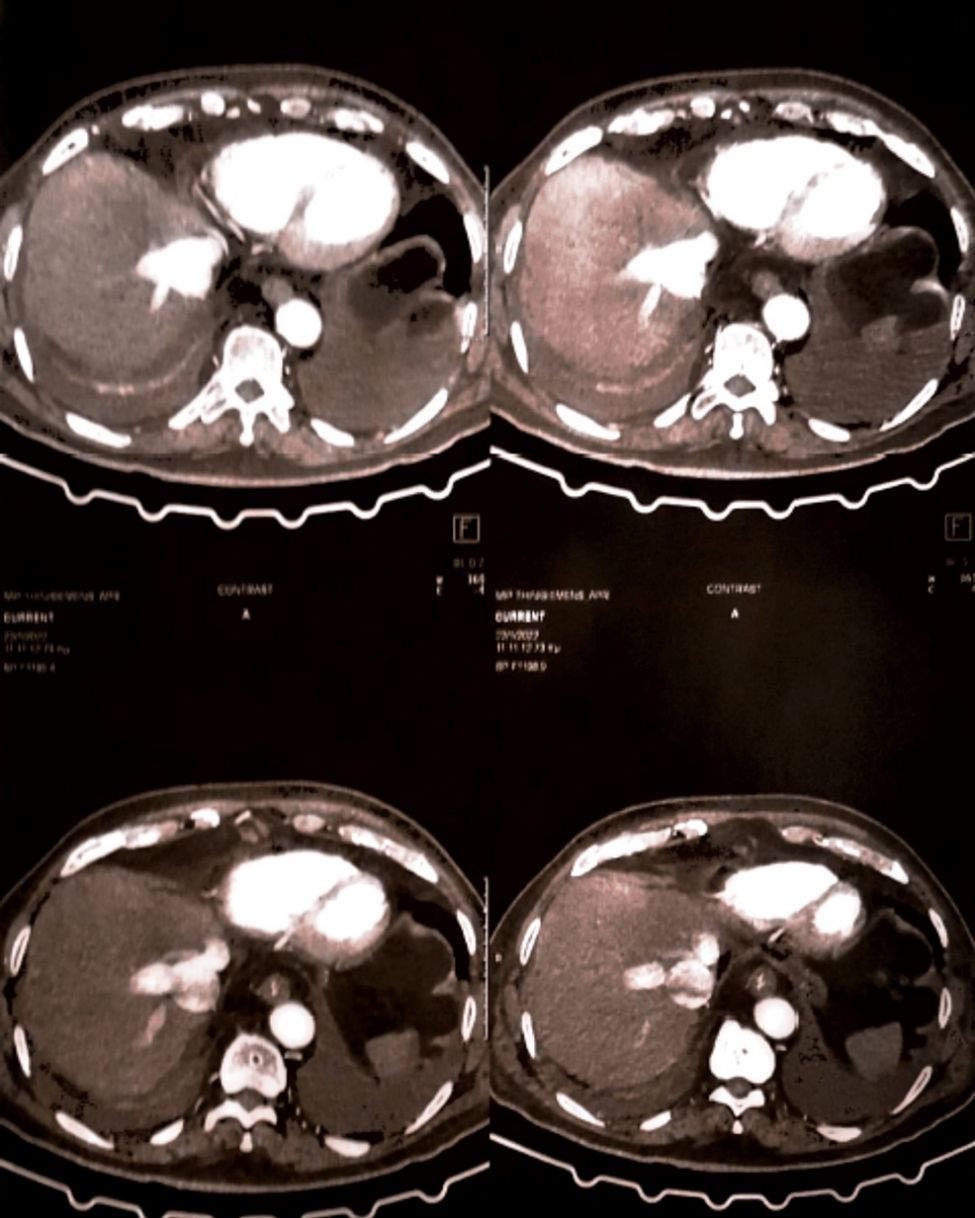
Computed tomography demonstrating mild liver enlargement, inferior vena cava dilatation, hepatic vein dilatation, and cardiac chamber enlargement.
